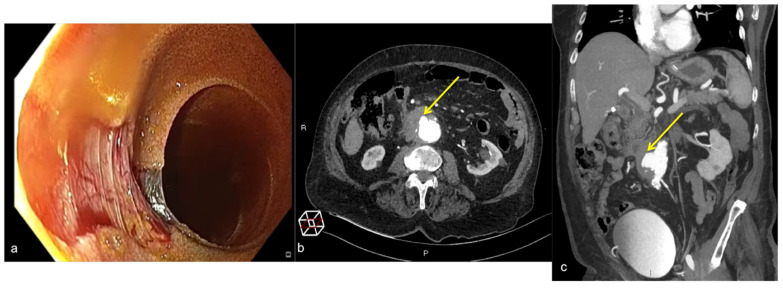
Hematochezia and abdominal pain with pulsatile mass. Fresh blood on colonoscopy without bleeding cause. Upper endoscopy (a) shows active pulsatile bleeding at the third duodenal portion. Axial CTA artery phase (b) and coronal MIP artery phase reconstruction (c) show a large outpouching from the right anterolateral wall of the abdominal aorta at the level of the third duodenal portion with loss of interface fat plane ((b,c) long arrows), The outpouching contains mural thrombosis. No air bubble is depicted in the aortic lumen or wall. No contrast extravasation into the duodenum is seen.
